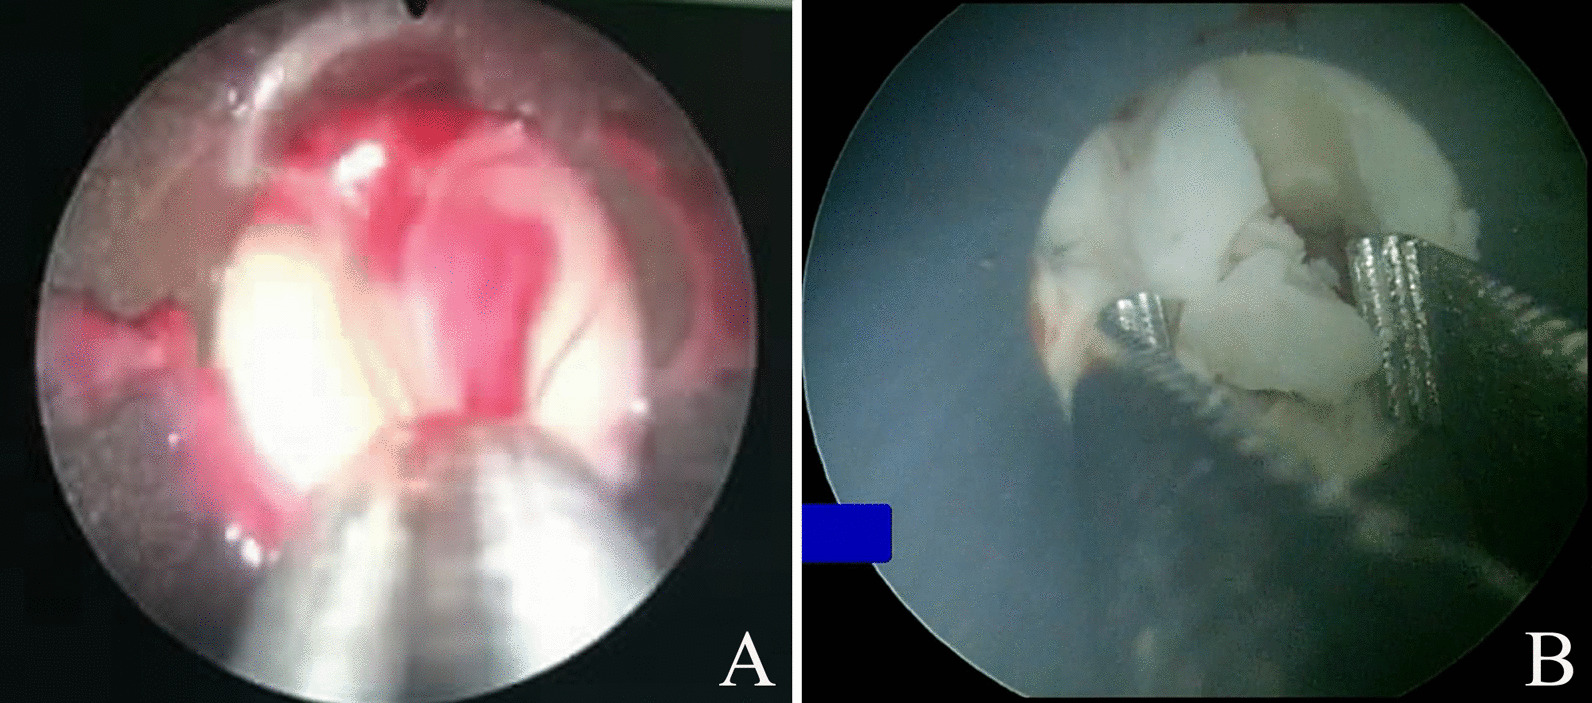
Intraoperative image that shows the percutaneous nephroscopy combined with ultrasound-guided negative-pressure suction for the treatment of perinephric abscess. A Negative-pressure suction was used to aspirate the pus. B Foreign body forceps were used to remove necrotic tissue
